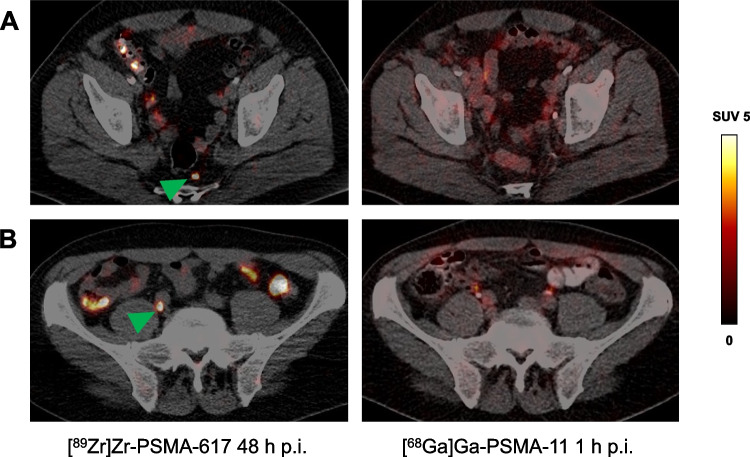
Transversal slice images showing presumed lymph node metastases of prostate cancer (green arrows) revealed by [^89^Zr]Zr-PSMA-617 PET/CT (left-hand column) but not identified on [^68^Ga]Ga-PSMA-11 PET/CT (right-hand column) in two patients (rows A and B, respectively). p.i., post-injection; SUV, standardized uptake value
